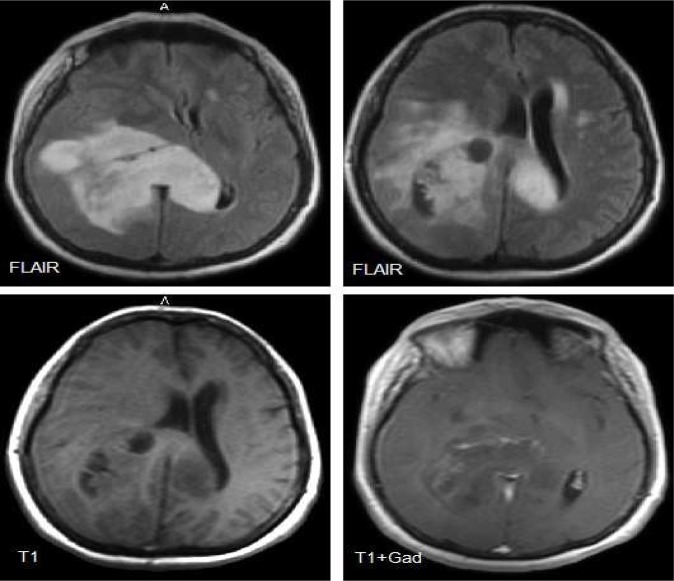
FLAIR images from different levels in axial brain MRI
